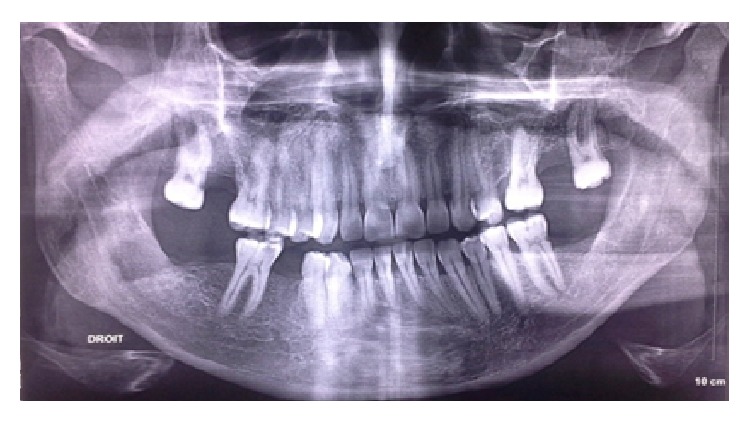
A 3-month postoperative panoramic radiograph.
